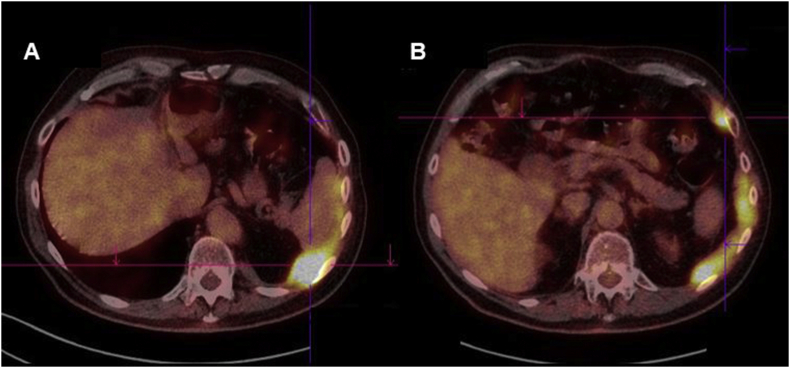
^18^F-fluorodeoxyglucose (FDG)- PET with computed tomography show a metabolic nodular localized (A) and diffuse (B) thickening of the left costo-diaphragmatic junction.
